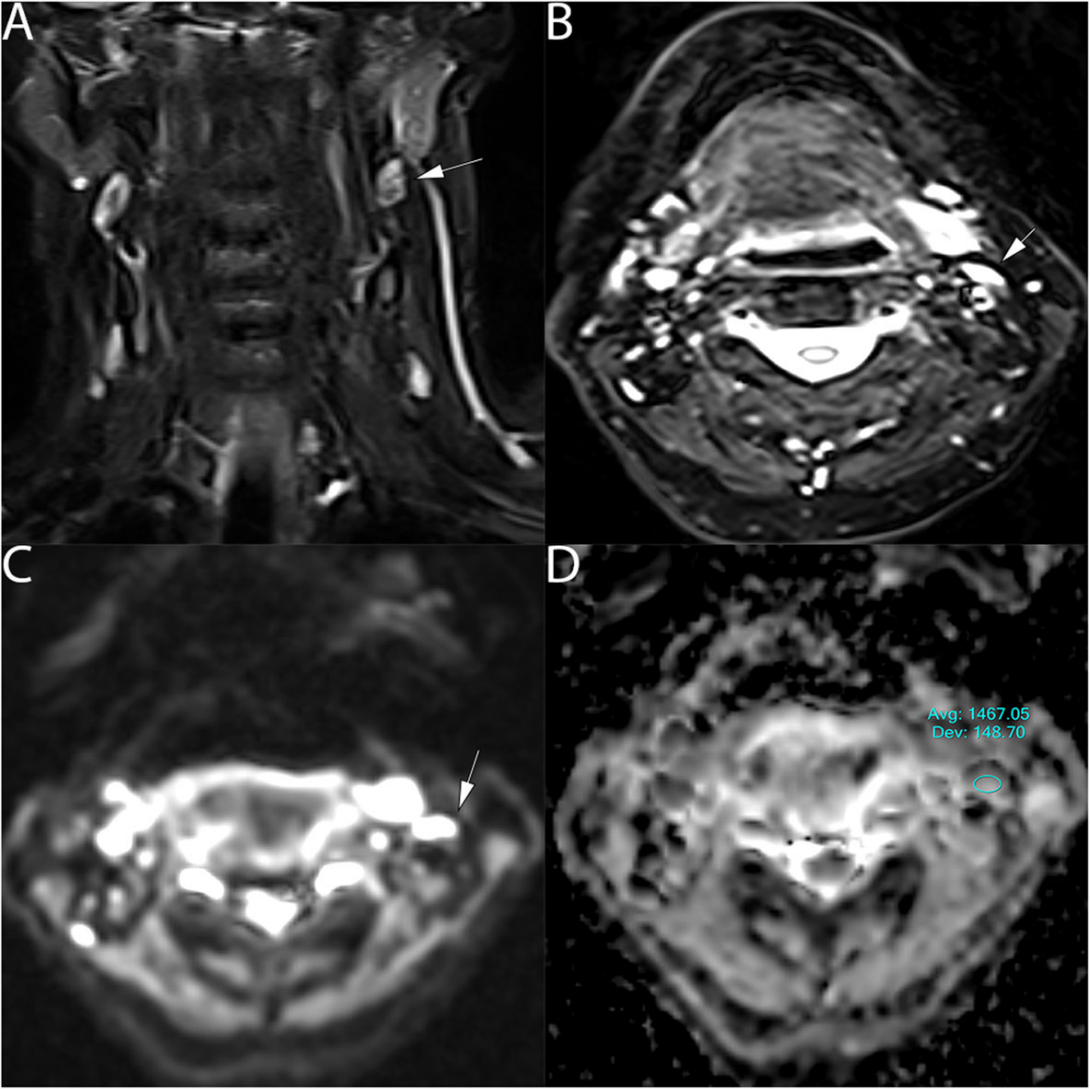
61 years old healthy male with normal lymph node in the left side of the neck. a T2 coronal show normal lymph nodes (arrow). b T2 axial view shows the axial extension of the left lymph node, (5x7mm), (arrow). c DWI show high signal at b value 800mm^2^/s (arrow) and (d) the ADC map on the targeted lymph node show an ADC value of (1.467 ± 0.15*10^− 3^ mm^2^/s) (arrow)
